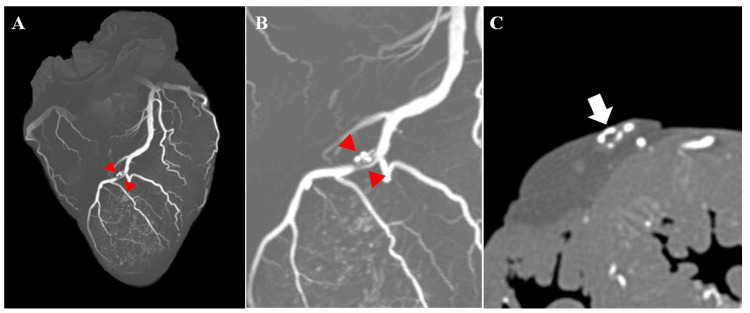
Coronary postmortem computed tomography angiography (PMCTA) images. (A) Maximum intensity projection (MIP) image in front view. A filling defect in the left anterior descending (LAD) artery is observed, as indicated by the red arrowheads. (B) Enlarged view of panel A. (C) Axial image of the LAD artery in the section corresponding to the red arrowheads in (A). A filling defect is evident, as indicated by the white arrows.
